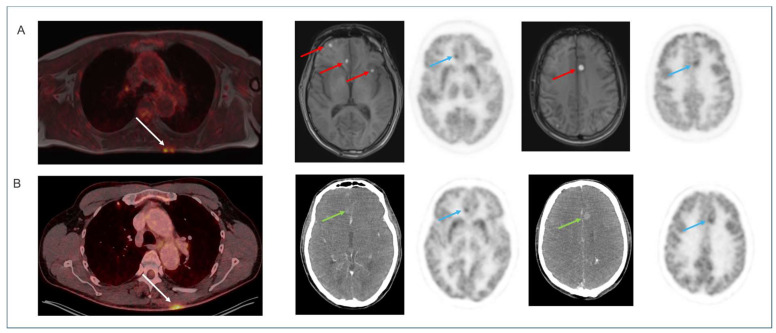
Total body imaging. Staging [^18^F]FDG PET in a patient with a surgically removed melanoma on the back. BIOGRAPH One PET/MRI (A) and PET/CT (B) from vertex to toe showed metabolic activity at the site of resection, interpreted as surgery related (white arrows), but also multiple metabolically active metastases in the brain (blue arrows) with contrast enhancement on CT (green arrows). Note that T1-weighted Dixon identified additional hyperintense foci (red arrows) reflecting melanin containing metastases not detected on CT or PET.
